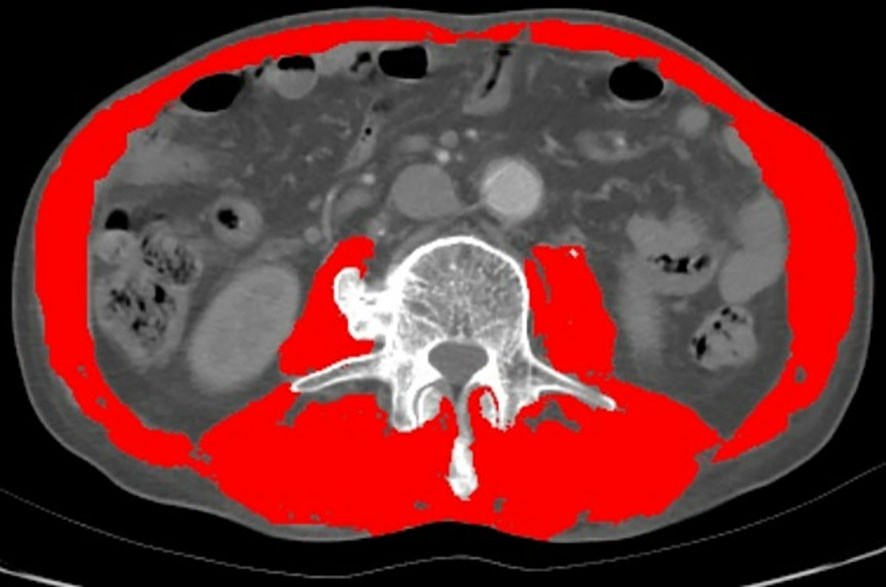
Evaluation of muscle quantity and quality at the third lumbar vertebra of the spine by computed tomography (CT). (The red part).
